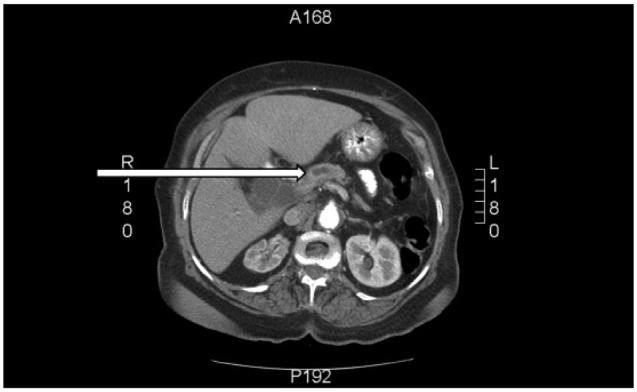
CT scan image of Case 1.
